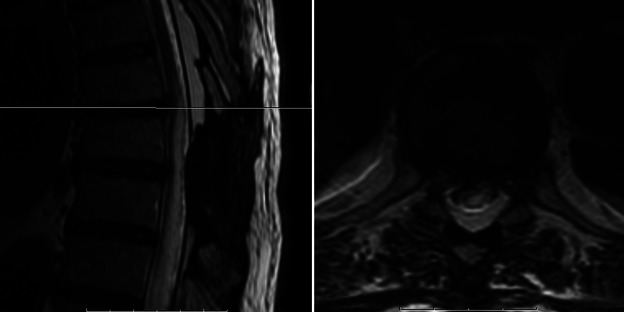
Preoperative focused T2-weighted sagittal (left) and corresponding axial (right) magnetic resonance (MR) images show significant cord edema terminating at the T7–8 disc, where right-sided herniation with cord compression and distortion is noted.
